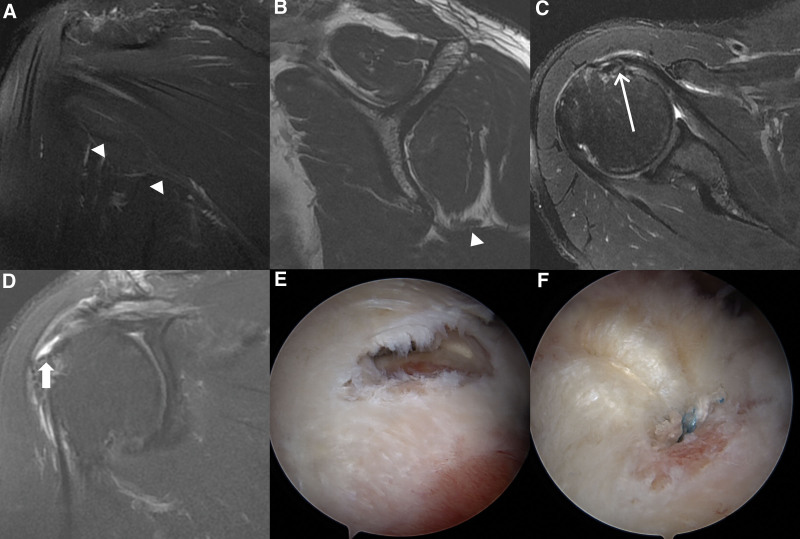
A 62-year-old male patient presented with recent limited ability to move his right arm and has been experiencing poorly localized pain in the posterior shoulder region for decades. The patient underwent arthroscopic rotator cuff repair and subsequent rehabilitation. At a 30-month follow-up, the patient was symptom-free. A. On fat-saturated T2-weighted (FST2W) oblique coronal image, decreased volume of the teres minor is suspected with minimal denervation edema (arrowheads). B. Oblique sagittal T1WI shows severe atrophy of the teres minor (arrowhead). C. Axial FST2W image reveals an articular side partial-thickness tear of the subscapularis and mild medial subluxation of the long head of the biceps tendon (long arrow). D. On FST2W oblique coronal image, a full-thickness tear of the supraspinatus is observed. E. Arthroscopic image confirms the full-thickness tear of the supraspinatus. F. Satisfactory arthroscopic rotator cuff repair is performed.
